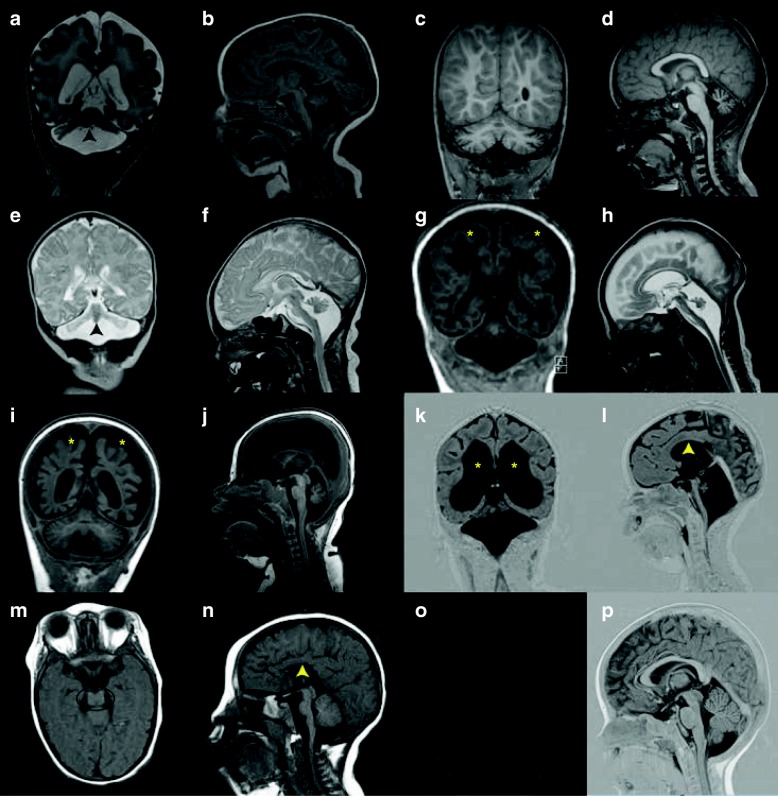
MR images in different PCH subtypes. a-b. Coronal (a) and midsagittal (b) image of severely affected PCH1B patient (age 3 weeks, homozygous for the p. G31A mutation in *EXOSC3*), showing severe hypoplasia of cerebellar hemisphere with relative sparing of the vermis (indicated by arrowhead). The ventral pons is reduced in size but not completely flattened (indicated by arrow). c-d. Coronal (c) and midsagittal (d) image of PCH1B patient (age 2,5 months) with milder *EXOSC3* mutations(the patient is homozygous for the p.D132A mutation). Cerebellar hypoplasia is very mild, with bilateral hyperintensities in the hili of the dentate nucleus. Size and shape of the ventral pons are normal. e-f. Coronal (e) and midsagittal (f) image of PCH2A patient (age 7 days, homozygous for the *TSEN54*, p.A307S mutation) with severe flattening of cerebellar hemispheres with relative sparing of the vermis (indicated by arrowhead) resulting in the ‘dragonfly’ configuration typical of PCH2A. This pattern is also seen in some PCH1 patients, as shown in 1A. The ventral pons is reduced in size but not completely flattened. g-h. Coronal (g) and midsagittal (h) image of PCH4 patient (age 6 weeks, compound heterozygous for the p. A307S mutation and a frameshift mutation in the *TSEN54* gene). Note severe flattening of pons and severe hypoplasia of the cerebellum without sparing of the vermis and profound supratentorial atrophy (indicated by asterisks). i-j. Coronal (i) and midsagittal (j) image of a PCH6 patient (age 6 months, compound heterozygous for mutations in the *RARS2* gene) with strikingly rapid progression of supratentorial atrophy (indicated by asterisks) and relatively slight atrophy of cerebellar hemispheres with normal pons and brainstem. k-l. Coronal (k) and midsagittal (l) image of a PCH7 patient (age 8 months, with biallelic mutations in the *TOE1* gene), showing severe pontocerebellar hypoplasia, equally affecting hemispheres and vermis. Note very thin corpus callosum and profound ventriculomegaly (indicated by asterisks and ^, respectively). m-n. Coronal and midsagittal image of a PCH9 patient (age 2 years, homozygous for a mutation in the *AMPD2* gene), showing characteristic ‘figure 8’ shaped brainstem and corpus callosum agenesis (indicated by a circle and arrowhead, respectively).o-p. Coronal and midsagittal image of a control
